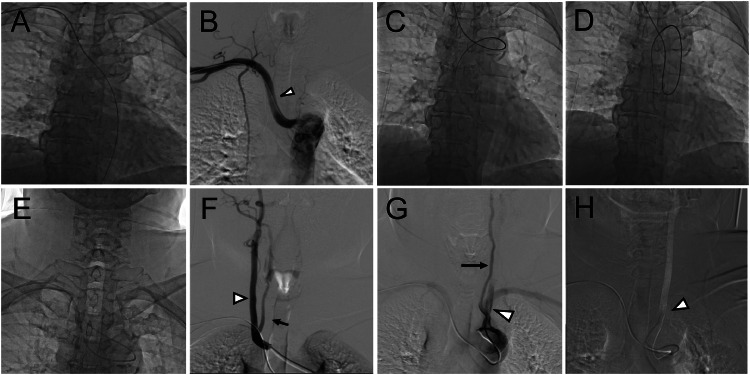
The radiographic imaging above (A–D) was obtained before accessing the carotid arteries. (A) X-ray image showing that the guidewire was exclusively advanced into the descending aorta. (B) Aortography revealing that the RSCA (arrowhead) is the final branch of the aortic arch. (C) X-ray image showing that the guidewire successfully advanced into the ascending aorta. (D) X-ray image subsequently shows the guidewire and catheter intolerantly herniating into the descending aorta. The radiographic imaging below (E–H) was obtained after selecting carotid arteries. (E) X-ray image showing that the catheter selects the right CCA while forming a Simmons curve. (F) Right CCA angiography showing a common trunk shared by the right CCA (arrowhead) and the right VA (black arrow). (G) Intra-aortic injection just distal to the origin of the right CCA showing, in order, the left CCA (arrowhead), the left VA originating directly from the aortic arch (black arrow), and the left subclavian artery. (H) DSA overlay showing that the right CCA is successfully selected by the catheter (arrowhead).
